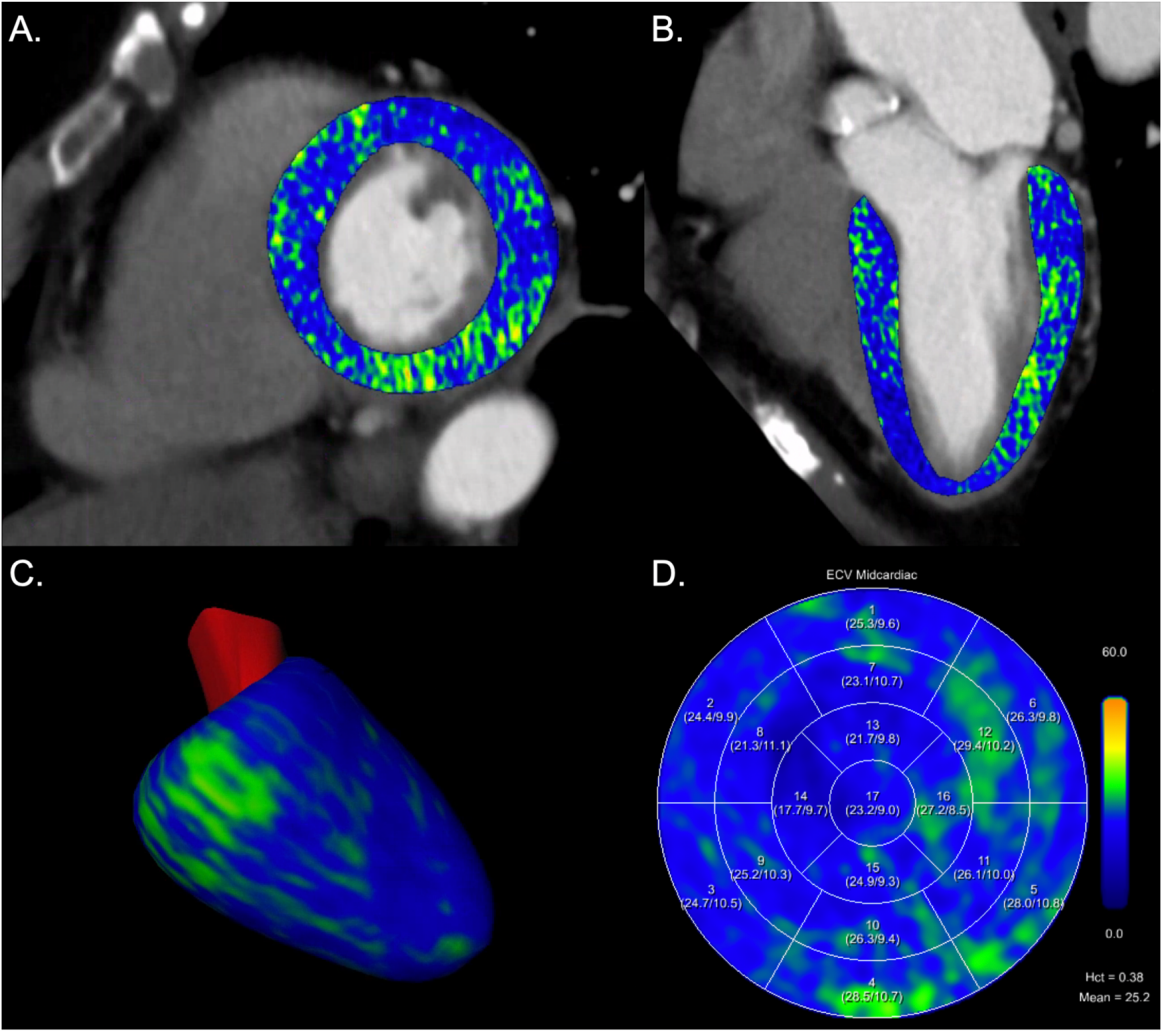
Myocardial extracellular volume (ECV) computation in a 72-year-old female patient with severe aortic stenosis. Images are displayed as short axis (A) and 2-chamber views (B) of extracellular maps calculated from the late enhancement scan superimposed on the coronary CT angiography images, 3D model of the left ventricle (C), and ECV polar map according to the 17-segment model of the American Heart Association (D). Blood hematocrit was 0.383 L/L resulting in a mean midmyocardial ECV of 25.2%; synthetic hematocrit was 0.402 L/L resulting in a mean midmyocardial ECV of 24.4%. Hct, hematocrit
